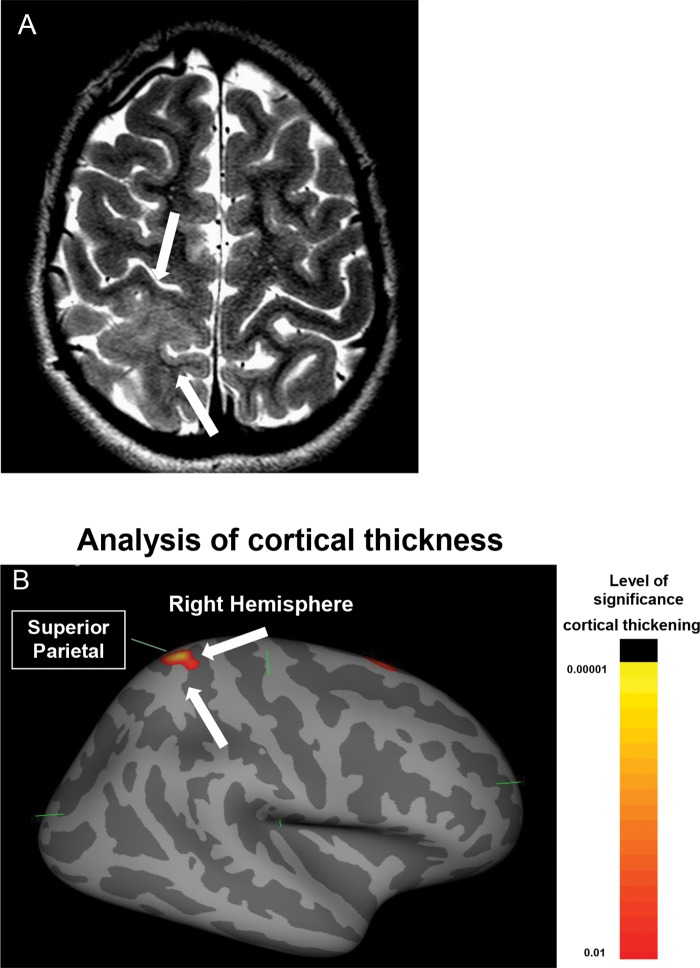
Focal cortical dysplasia type IIB of Palmini in a 10-year-old girl. A) An axial T2-weighted image demonstrating a focal thickening of the right parietal cortex (white arrows). B) An automated analysis of cortical thickness generated by Freesurfer using a volumetric T1-weighted image. These measurements clearly show a significant focal area of cortical thickening in the right superior parietal region that corresponds to the lesion observed in the T2-weighted sequence.
